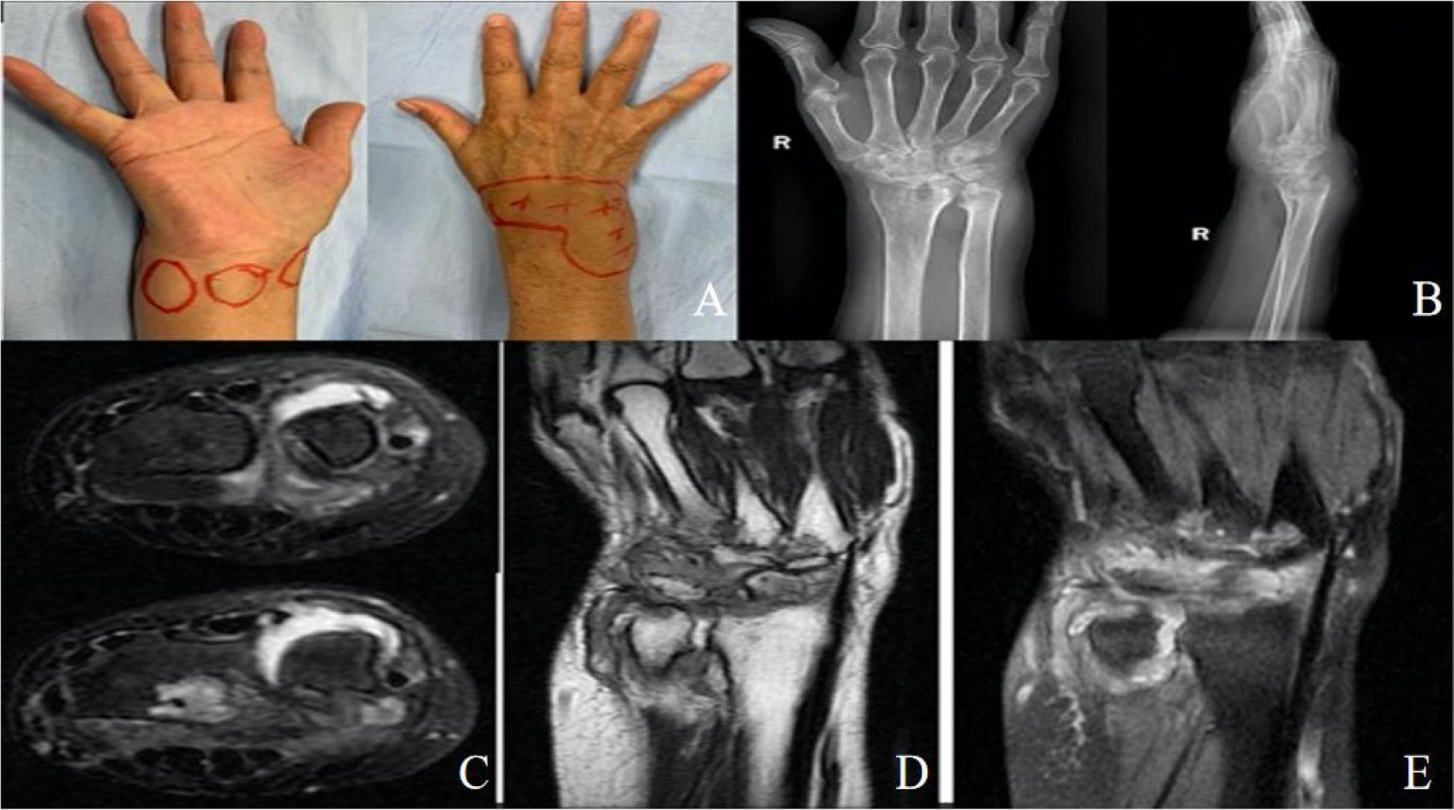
A 49-year-old male patient who received conservative treatment. A. Swelling can be observed on the palmar and dorsal side of the right wrist. B. Anteroposterior and lateral X-ray of the right wrist revealed malalignment of the wrist with an osteolytic lesion of the distal radius, distal ulna, and the proximal carpal bones. C. MRI images of the right hand showed osteolytic lesions in the distal ulna and carpal bones, and extensive necrotic degeneration of the synovium and surrounding soft tissues of the wrist joint
